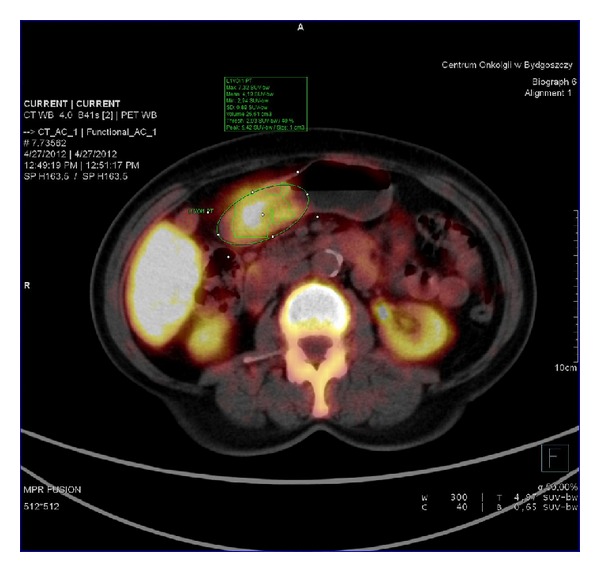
^18^FLT-PET in patient with adenocarcinoma tubulare G2 (G3). SUV_max⁡_ = 7.32.
